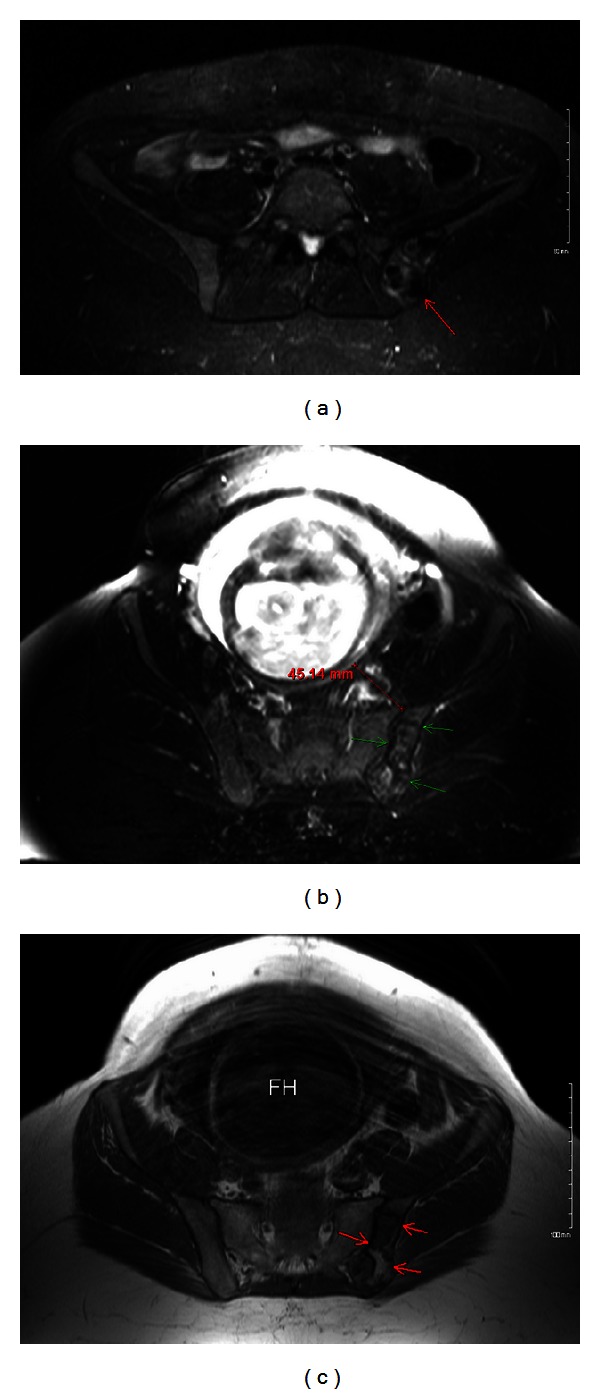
(a) Short tau inversion recovery (STIR) MR image showing lesion with gadolinium contrast. (b) T2 fat-saturated MR image without contrast. Green arrows indicate the bone lesion. The fetal head (FH) is also seen 4.5 cm away from lesion. (c) T1-weighted MR image without contrast. Red arrows indicate the bone lesion. The fetal head (FH) is also seen.
